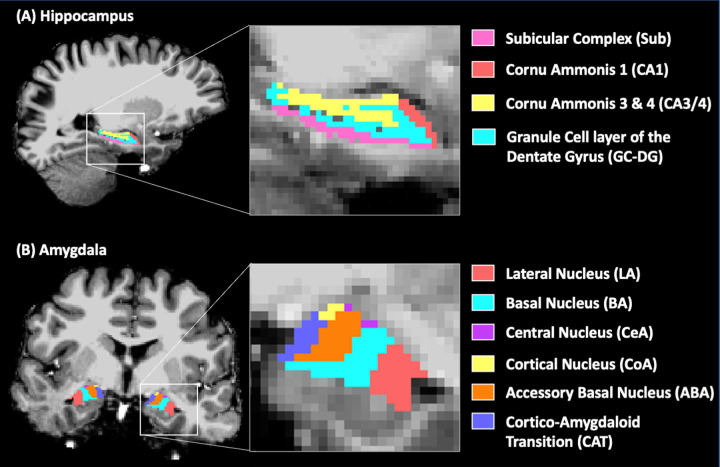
Hippocampus and amygdala subregion segmentation. A single-subject hippocampus (A) and amygdala (B) subregions FreeSurfer segmentation overlaid on the T1-weighted image.
